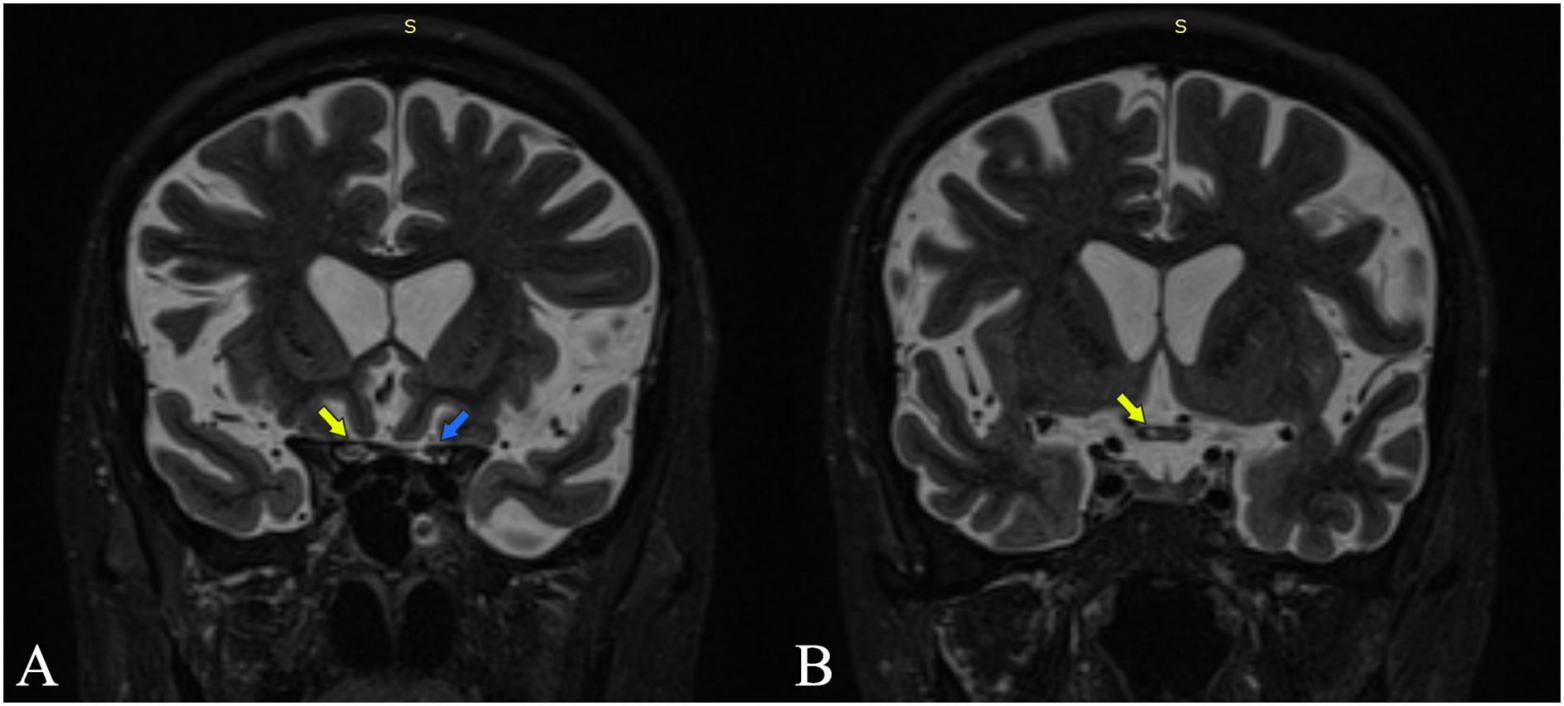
Coronal MRI STIR sections are at the intracranial optic nerves (A) and optic chiasm (B). Yellow arrows point out increased STIR signal intensity at the level of the right optic nerve and the right side of the chiasm. The blue arrow points out the left optic nerve with normal signal intensity.
